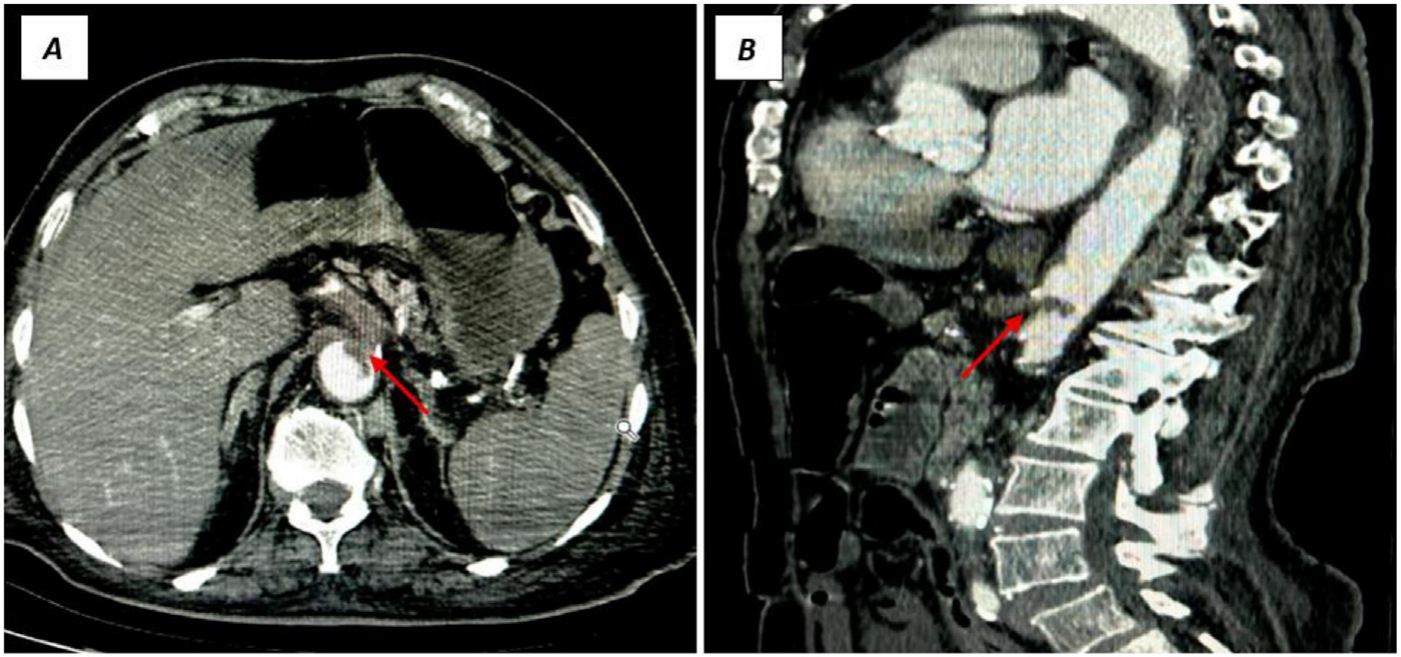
Abdominal CT angiography showing acute occlusion of the celiac trunk. (A) Axial view, (B) Sagittal reconstruction, demonstrating absence of opacification of the celiac trunk (red arrows).
